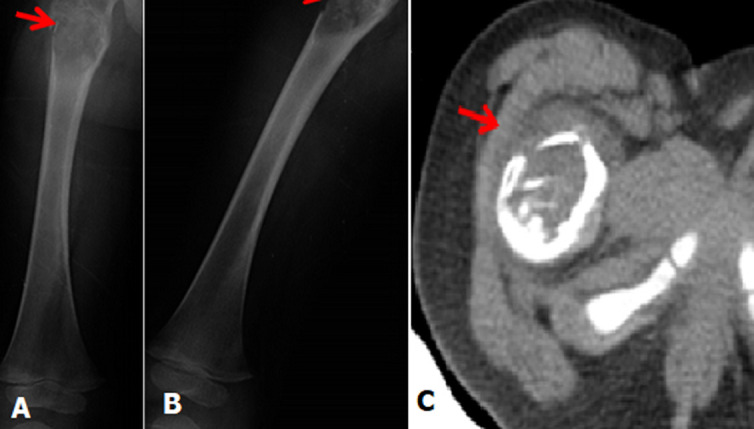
A) frontal femur X-ray; B) lateral femur X-ray; C) right hip CT scan: showing an eccentric osteolytic metaphyseal-diaphyseal lesion disrupting the cortex without periosteal reaction at the level of the right femur
